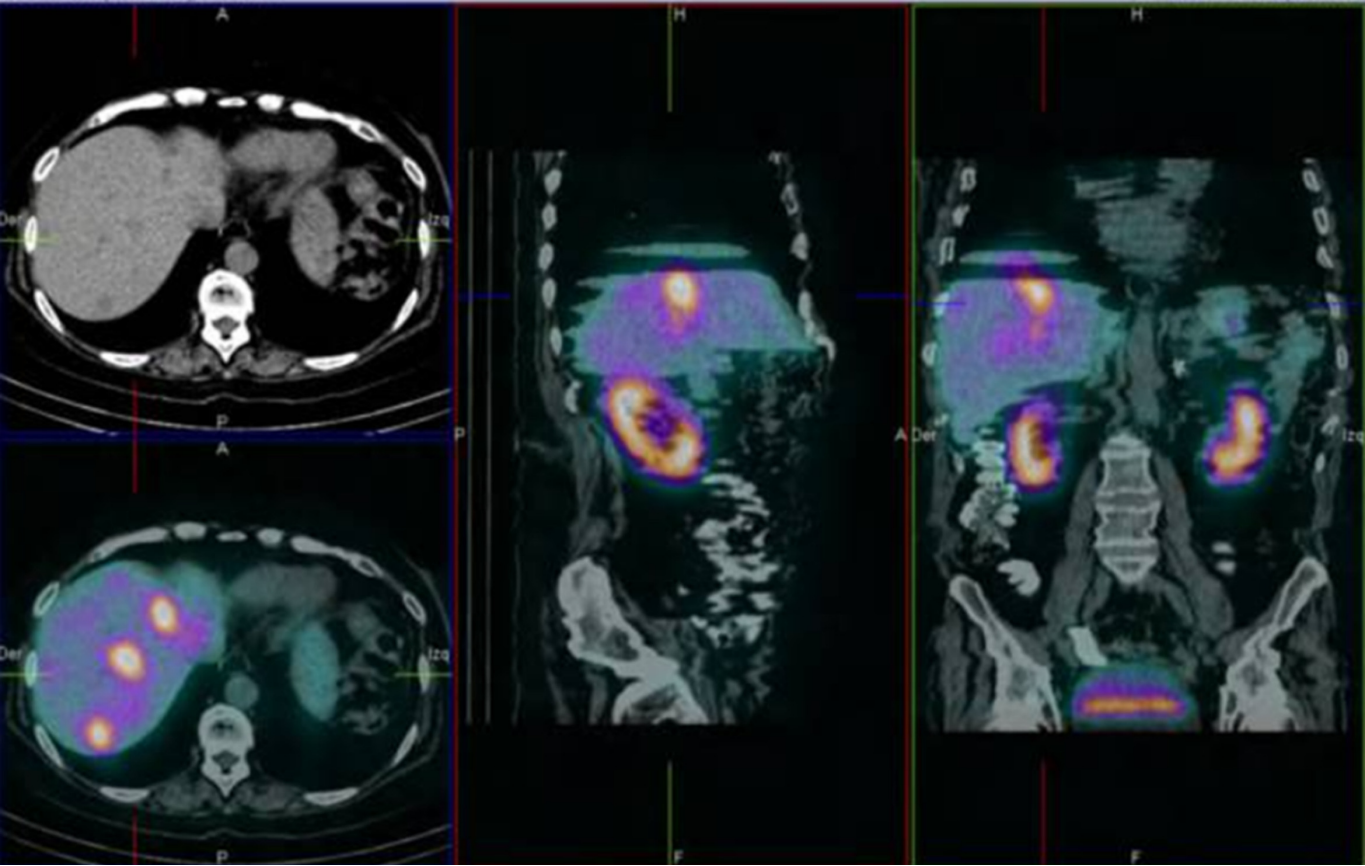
SPECT‐CT showing liver metastases secondary to neuroendocrine ACTH‐secreting pancreatic tumor.
